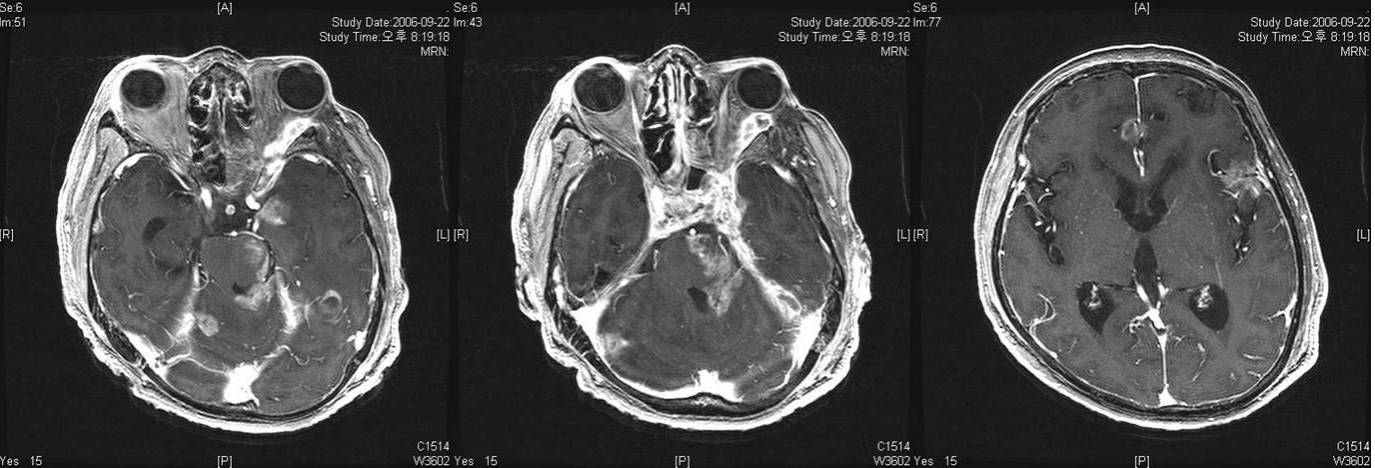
Follow-up brain MR images revealing a slightly decreased tumor size extending to orbital, cavernous sinus, infratemporal fossa, and clivus. However, small nodular enhancing lesion on the interhemispheric fissue and superior cerebellar peduncle, and leptomeningeal seeding is suspected.
